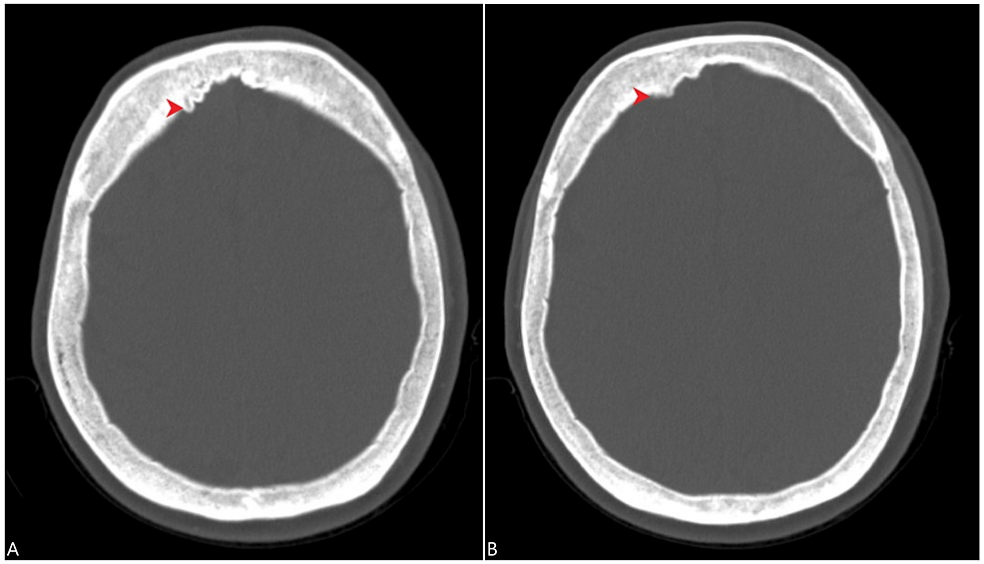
CT scan of the head demonstrated hyperostosis of the right frontal bone (red arrowheads) in the bone window, as shown here in two different sections (A and B). CT: computed tomography
